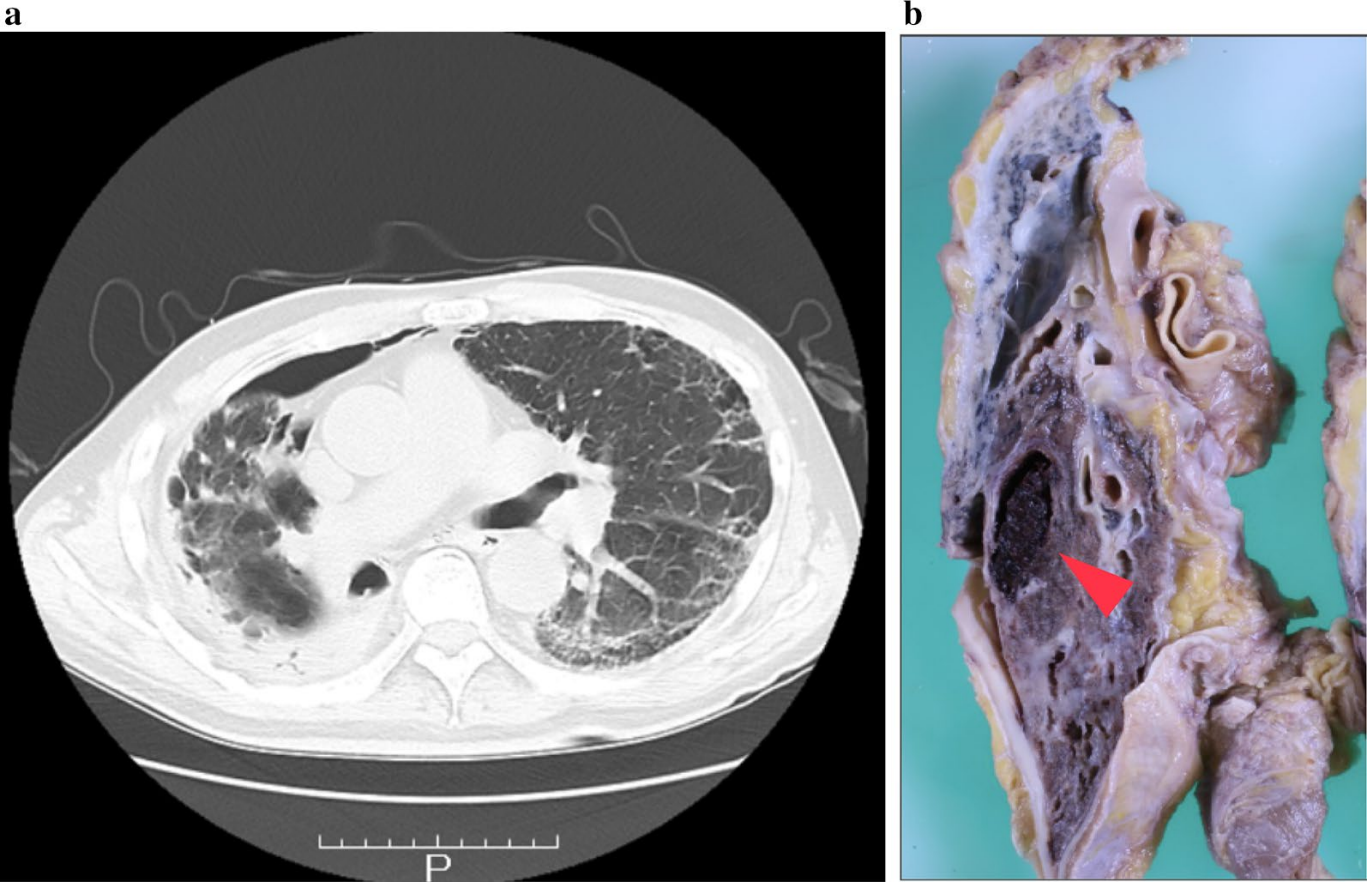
Chest CT and lung autopsy of case 1. a Chest CT shows bilateral emphysema and pneumothorax. b Lung autopsy shows many cysts and one cyst in the right middle lobe contains a relatively new hemorrhage (red arrowhead)
